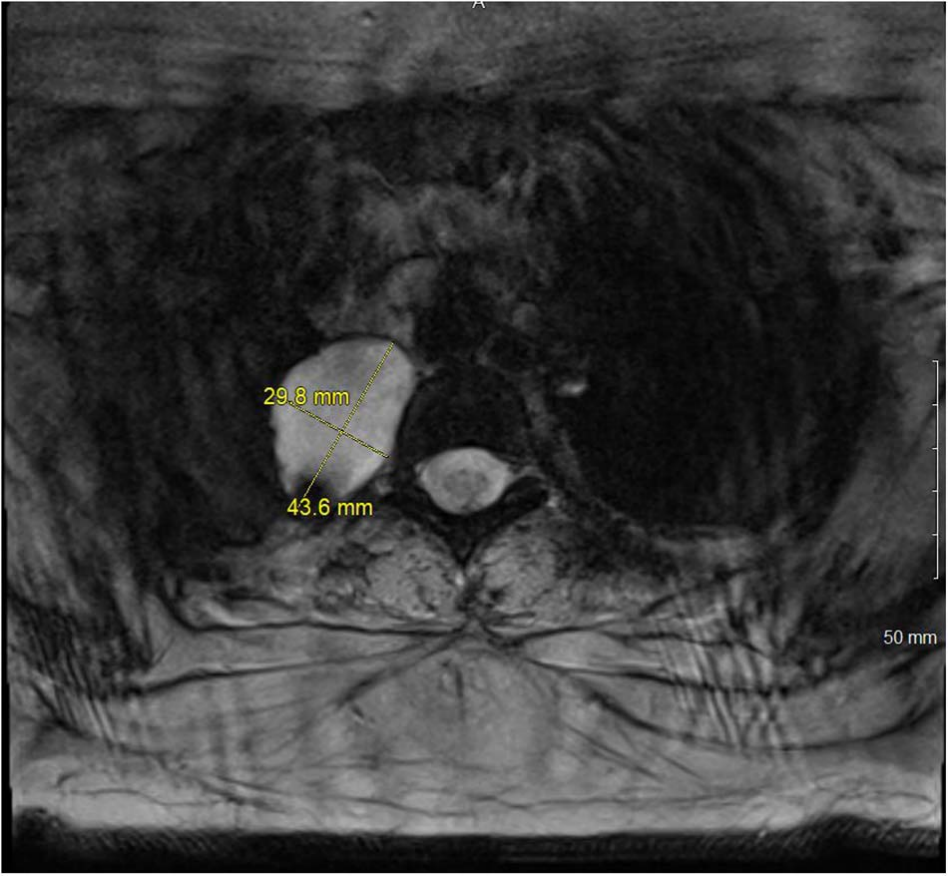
T2 MRI transverse view of T2-T3 paravertebral mass.
